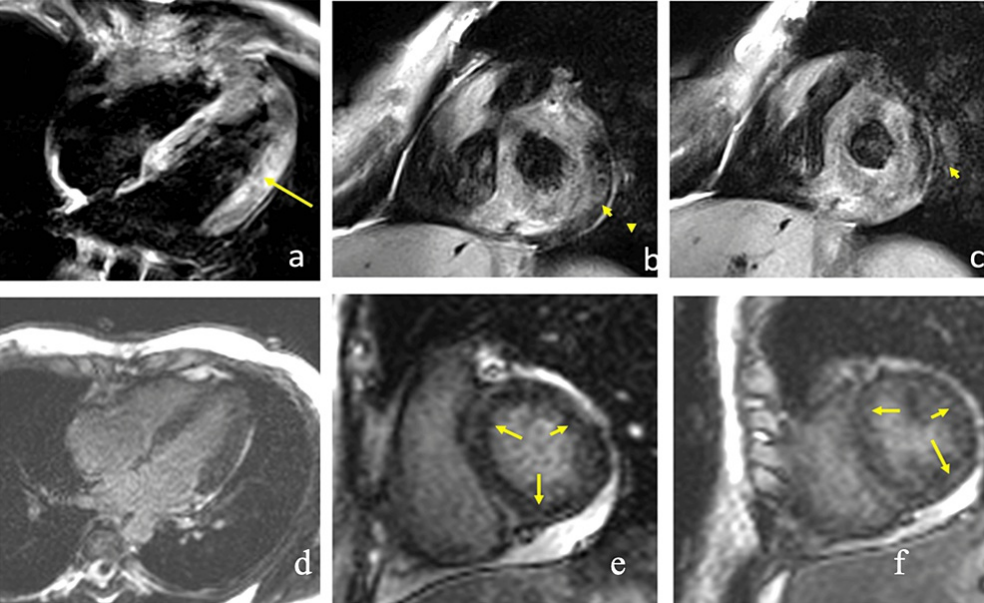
Cardiac MRI Four-chamber T2-weighted sequence (a), basal third short axis (b), and half (c). The arrow points to the lateral hyperintensity of the middle third and the arrowhead to the pericardium adjacent to the inferior and lateral wall. In d-f, the inversion-recovery sequence in the same planes as a-c is seen; the arrow indicates the global intramyocardial late enhancement of the left ventricle, predominantly basal.
